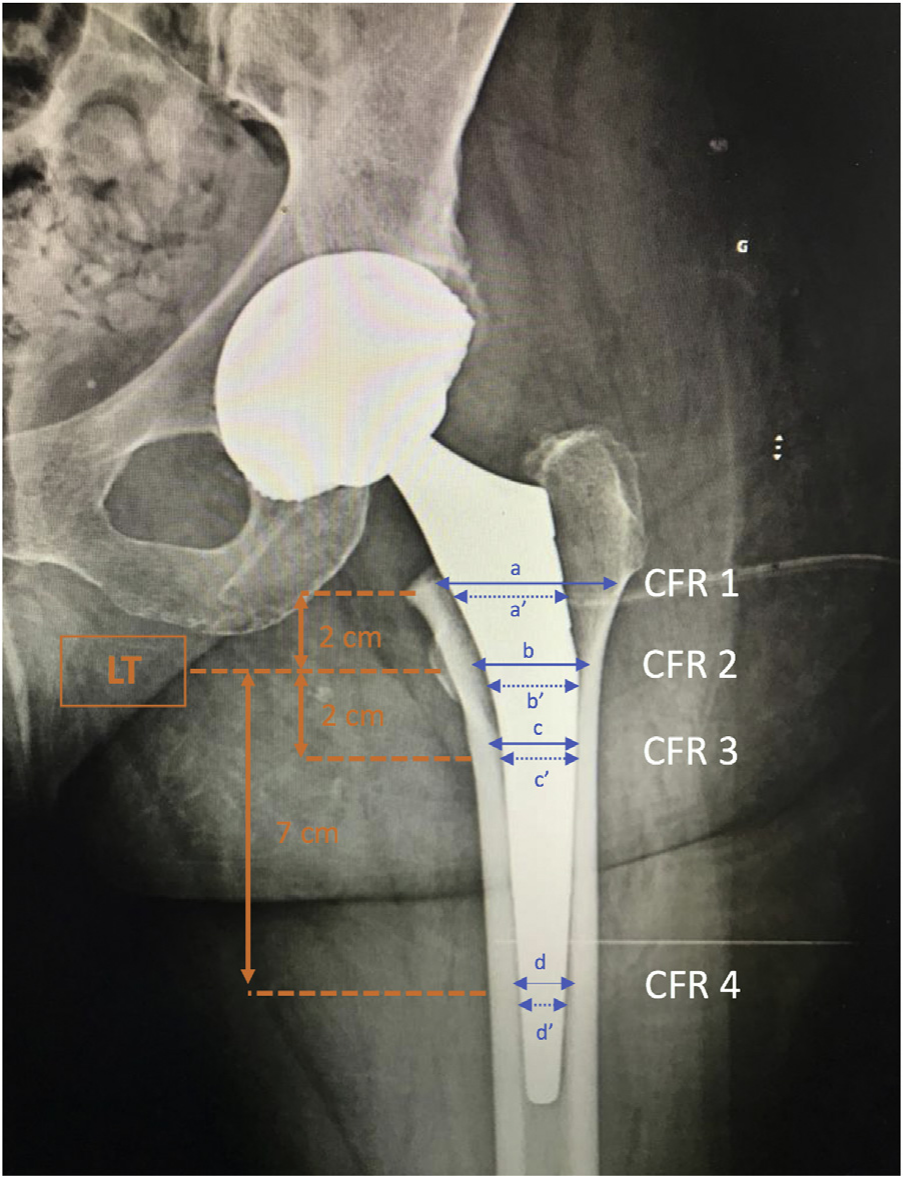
Dimensions of the implant were evaluated by radiographic measurement of the canal fill ratio (CFR) at 4 different levels on the postoperative radiograph: at the level of the lesser trochanter, 2 cm above and below the lesser trochanter and 7 cm below the lesser trochanter.
